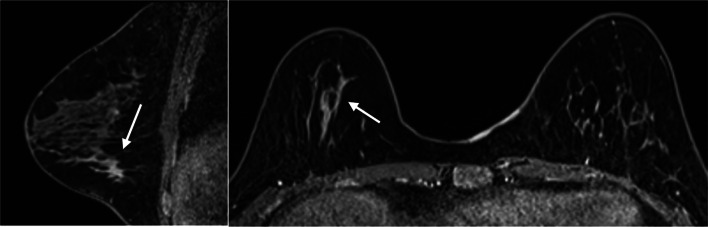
Contrast-enhanced prone MRI depicted a region with NME in the right breast (arrow)
